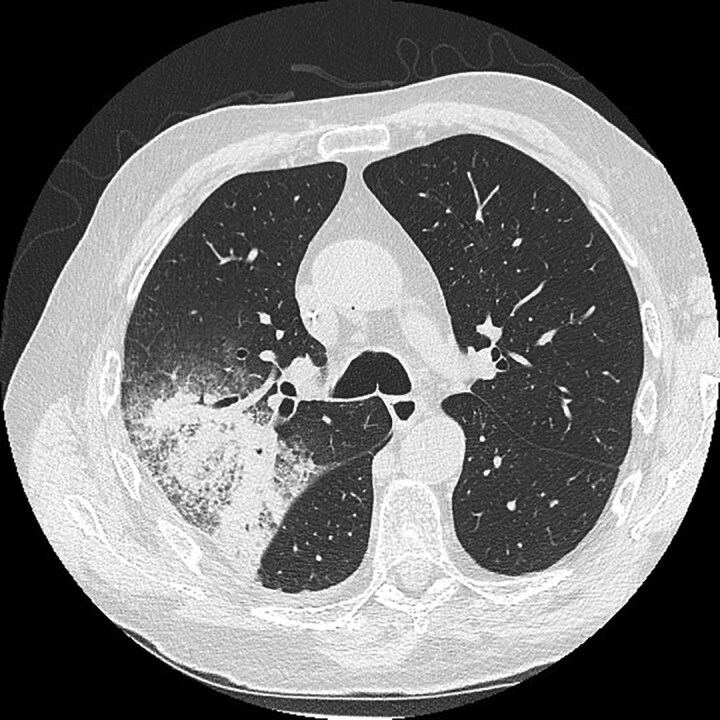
CT scan image (axial view) of a reverse halo sign. This is defined as a central area of ground-glass opacification surrounded by a denser peripheral area of consolidation. Courtesy of Professor Samantha Ellis, Department of Radiology, Alfred Health, Melbourne.
